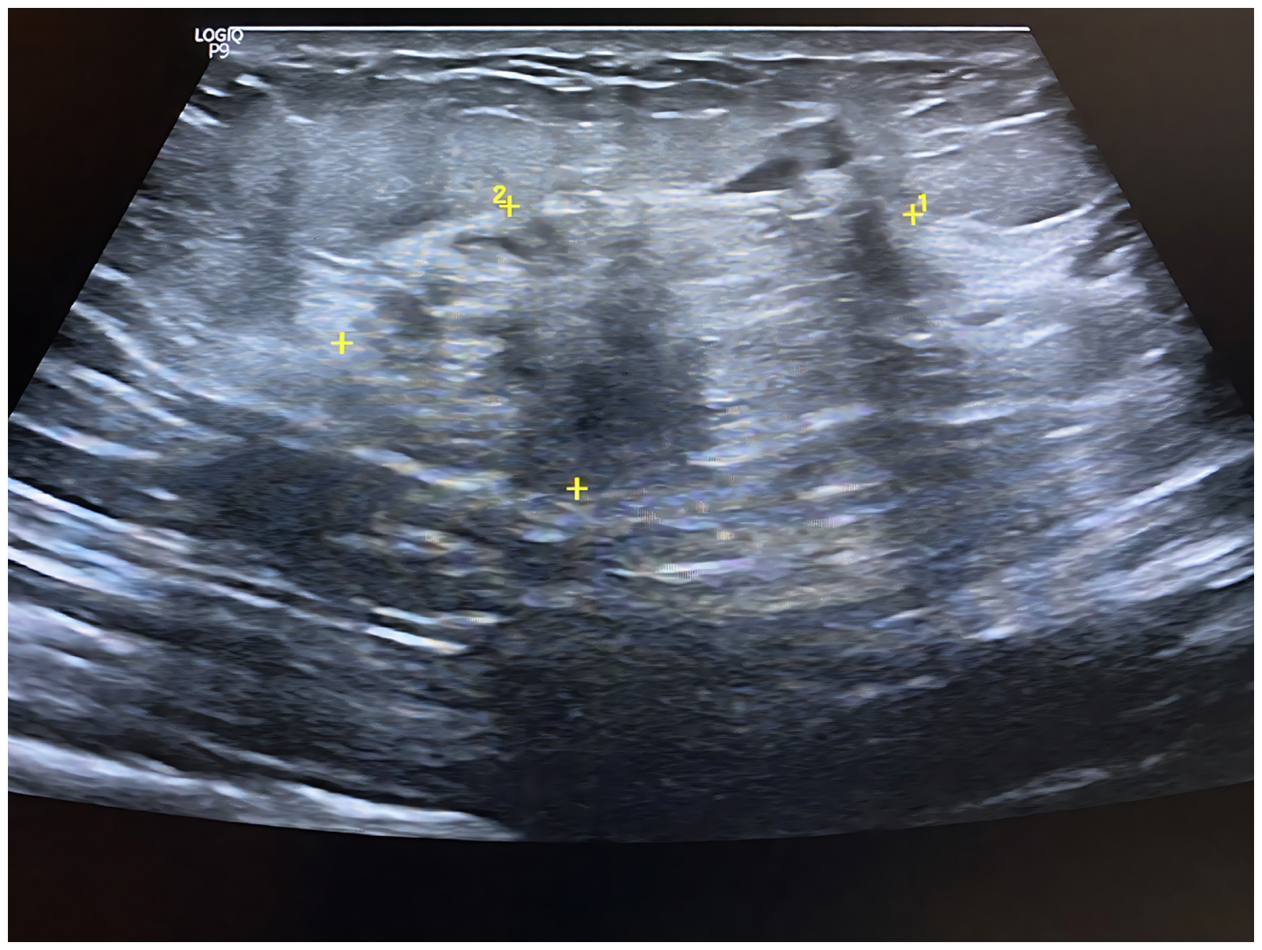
Grayscale ultrasound of the right axilla demonstrating accessory breast tissue with heterogeneous parenchymal echotexture and mild edema, consistent with mastitis. The numbers ‘1’ and ‘2’ designate the two orthogonal caliper sets used to measure the long- and short-axis of the lesion, respectively; the yellow crosses indicate the caliper endpoints. The recorded dimensions are 36x9 mm (long x short)
